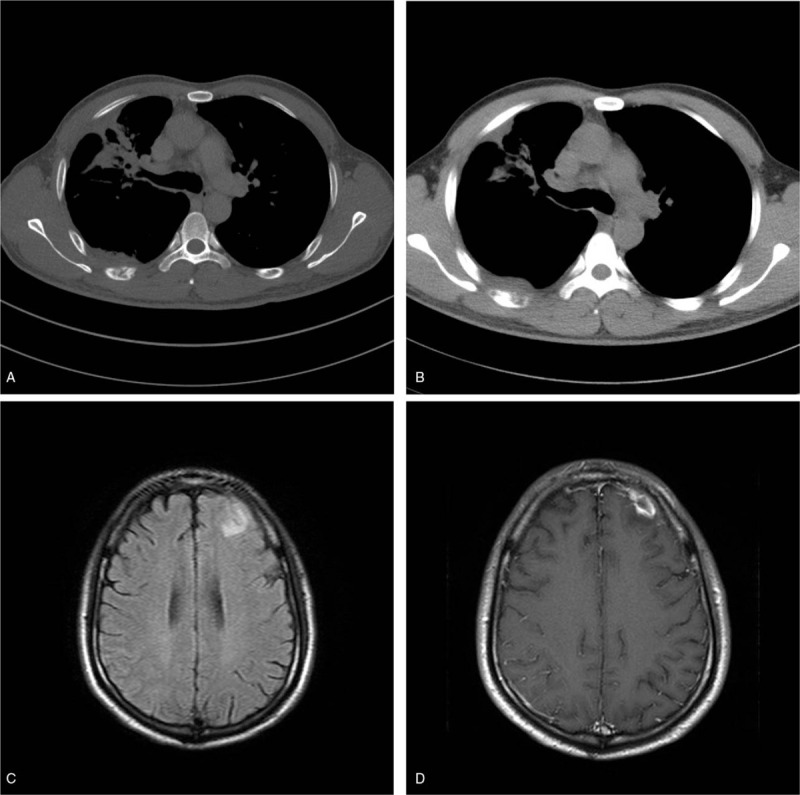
Male, 37 years old, recurrent cough and fever for 1 month. The new lesions appear in the right upper lung in massive opacities and cord-like shadows with bone destruction (A) and soft tissue mass (B) in the right sixth rib. MRI shows a patchy high signal in the left frontal lobe on T2WI/FLAIR (C). The lesion shows an annular enhancement (D).
